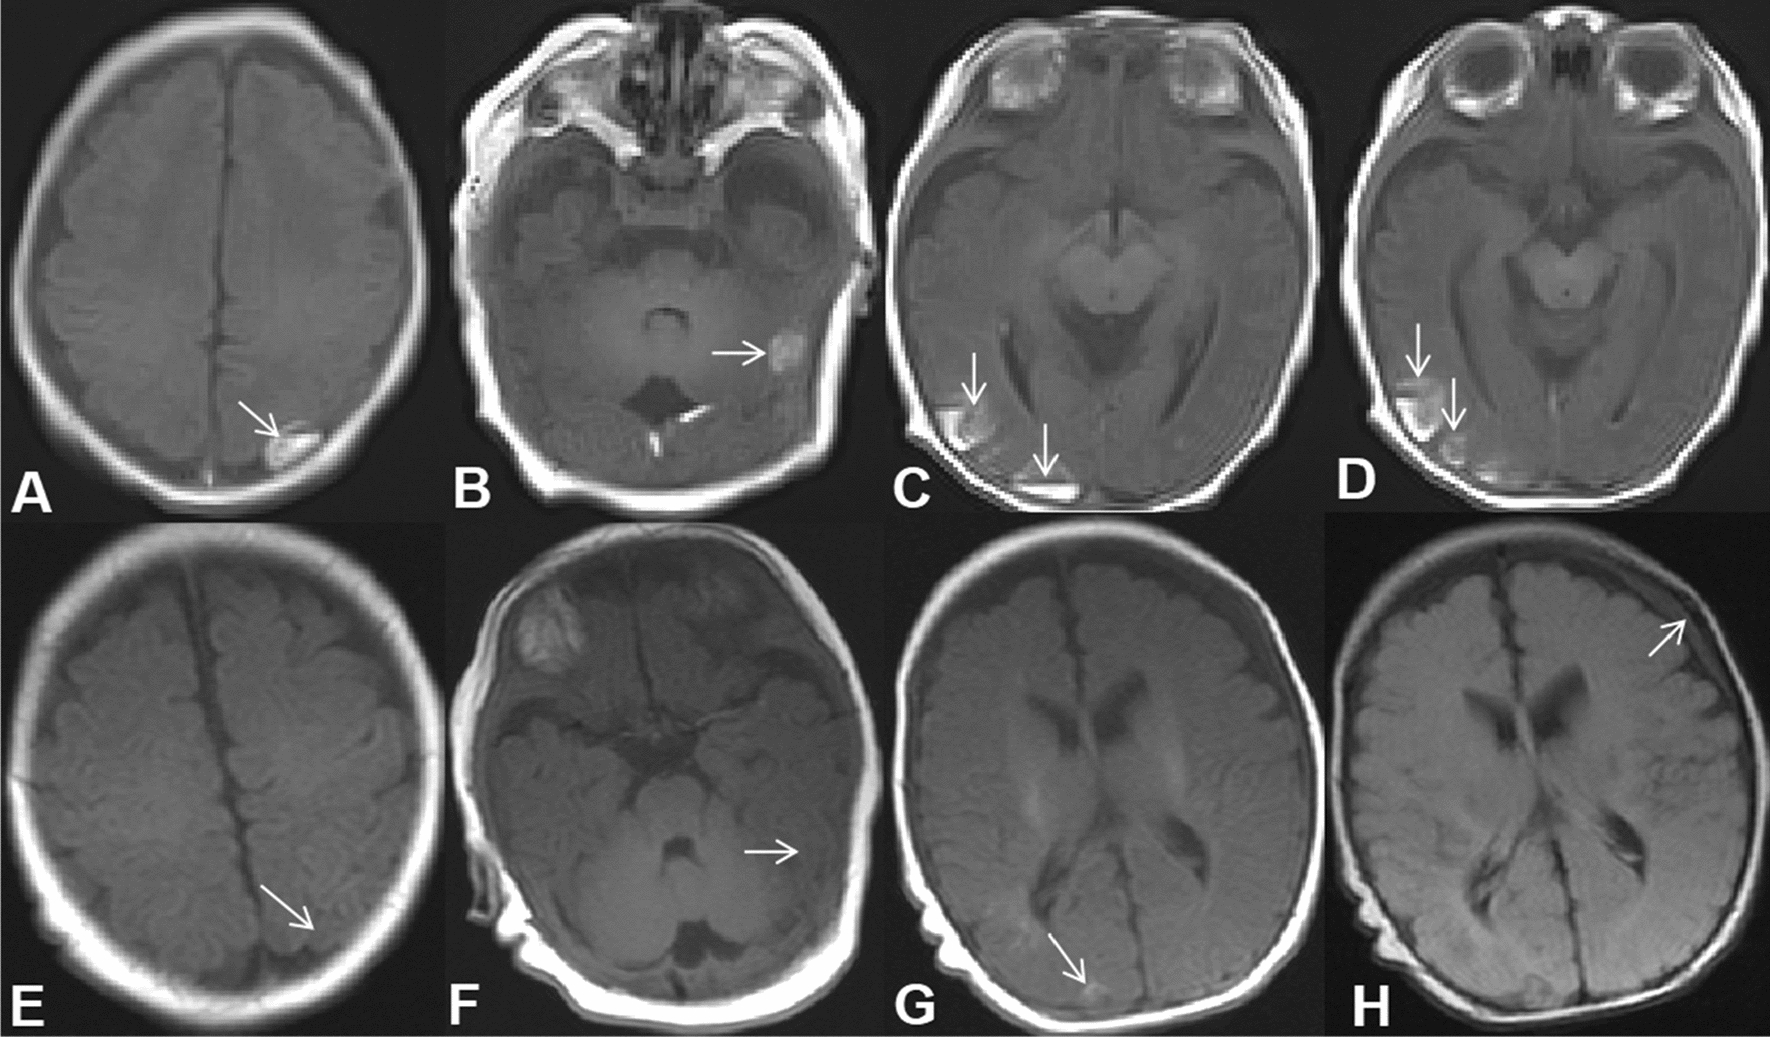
Magnetic resonance imaging (MRI) in newborn (A–D All images are T1-weighted) and follow-up (E, F T1-weighted images; G, H T2 flair images) 2 months later. A Left parietal haematoma. B Left temporal lobe haematoma. C, D Multiple haematoma in right occipital lobe. E The haematoma in the left parietal lobe has been absorbed. F The haematoma in the left temporal lobe has been absorbed. G The right occipital haematoma was absorbed more obviously than before. H Chronic subdural haematoma of left frontal region
